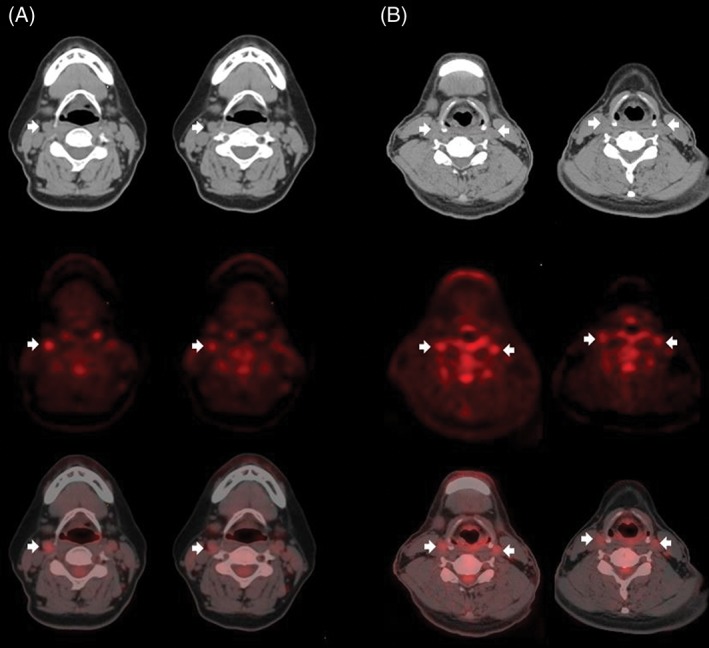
Index vessel ^18^FDG uptakes for a patient treated with (A) fimasartan or (B) amlodipine (arrows): (top) representative CT, (middle) ^18^FDG‐PET, (bottom) ^18^FDG‐PET/CT images at (left) baseline and (right) 6‐month follow‐up. ^18^FDG uptakes markedly decreased at the 6‐month follow‐up. CT, computed tomography; ^18^FDG, ^18^F‐fluorodeoxyglucose; PET, positron emission tomography
